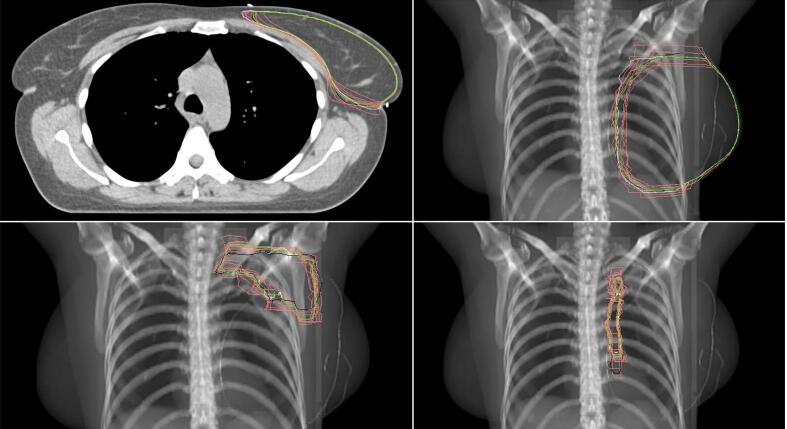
Axial CT image and topogram showing CTV breast, CTVN and CTV-IMN for all included centers. Raystation’s CTV is black, MVision’s CTV white and the Mean-CTV-structure green. (For interpretation of the references to colour in this figure legend, the reader is referred to the web version of this article.)
